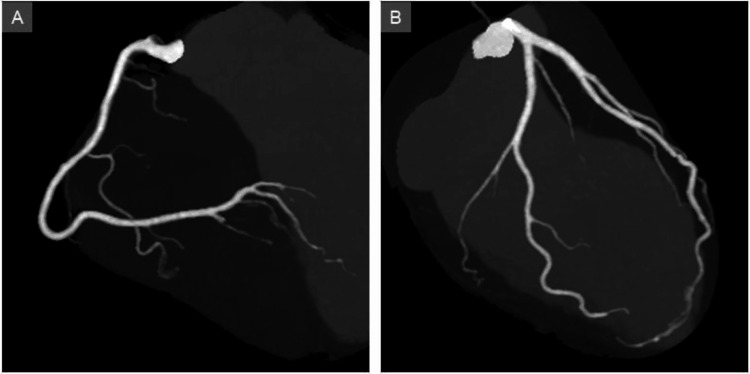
Computed tomography angiography Computed tomography angiography revealed no significant stenosis in either the right or the left coronary artery (A and B).
